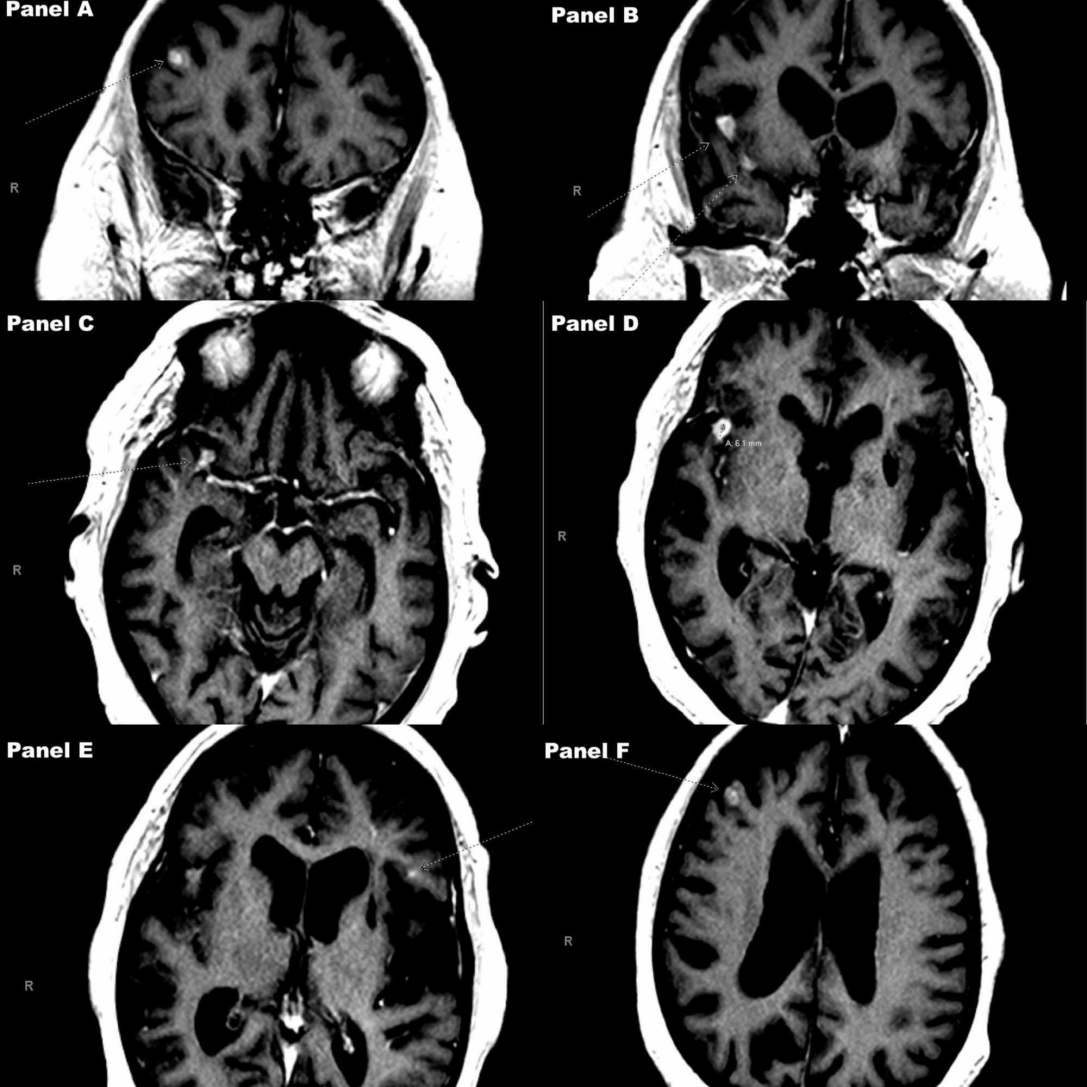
Diffusion weighted images (DWI) on MR of the brain with and without gadolinium contrast Magnify image to view arrows pointing out the intraparenchymal lesions themselves. Panels A and B show coronal cuts. Panels C through F show axial cuts.
